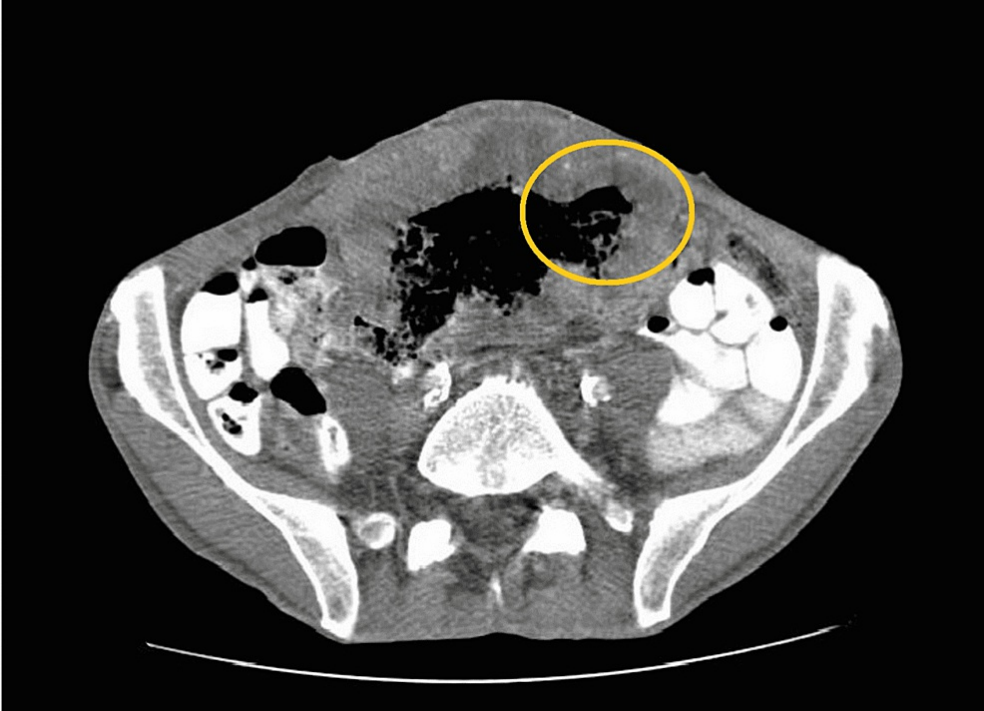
Coronal sections of abdominal CT images revealed a mass of the abdominal wall invading the colon (yellow circle).
